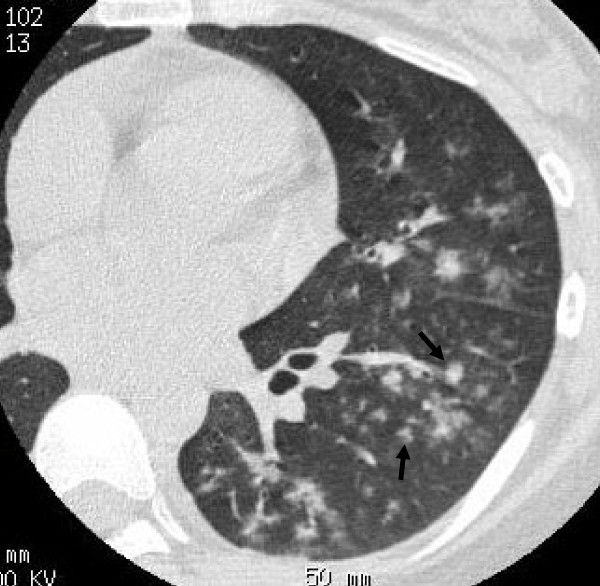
24-year-old man with *M. pneumoniae *pneumonia. CT shows centrilobular nodules (tree-in-bud appearance, arrows). Bronchial wall thickening is also seen.
